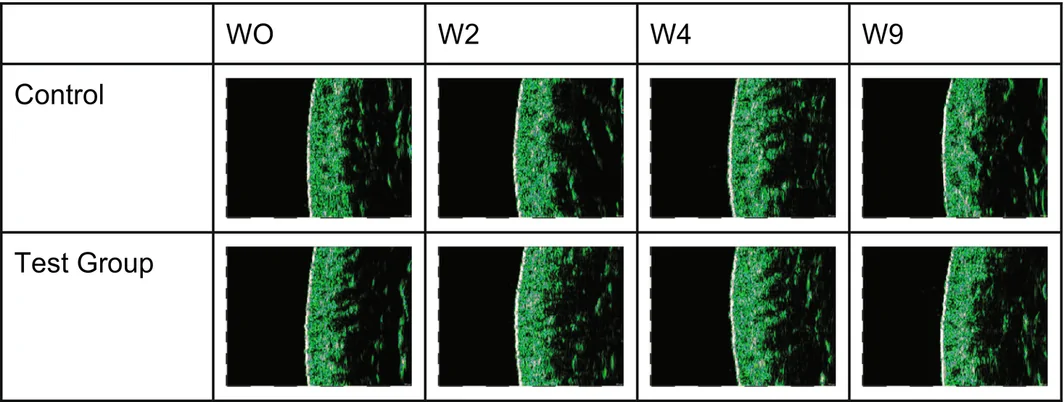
Dermal ultrasound echogenicity images.
